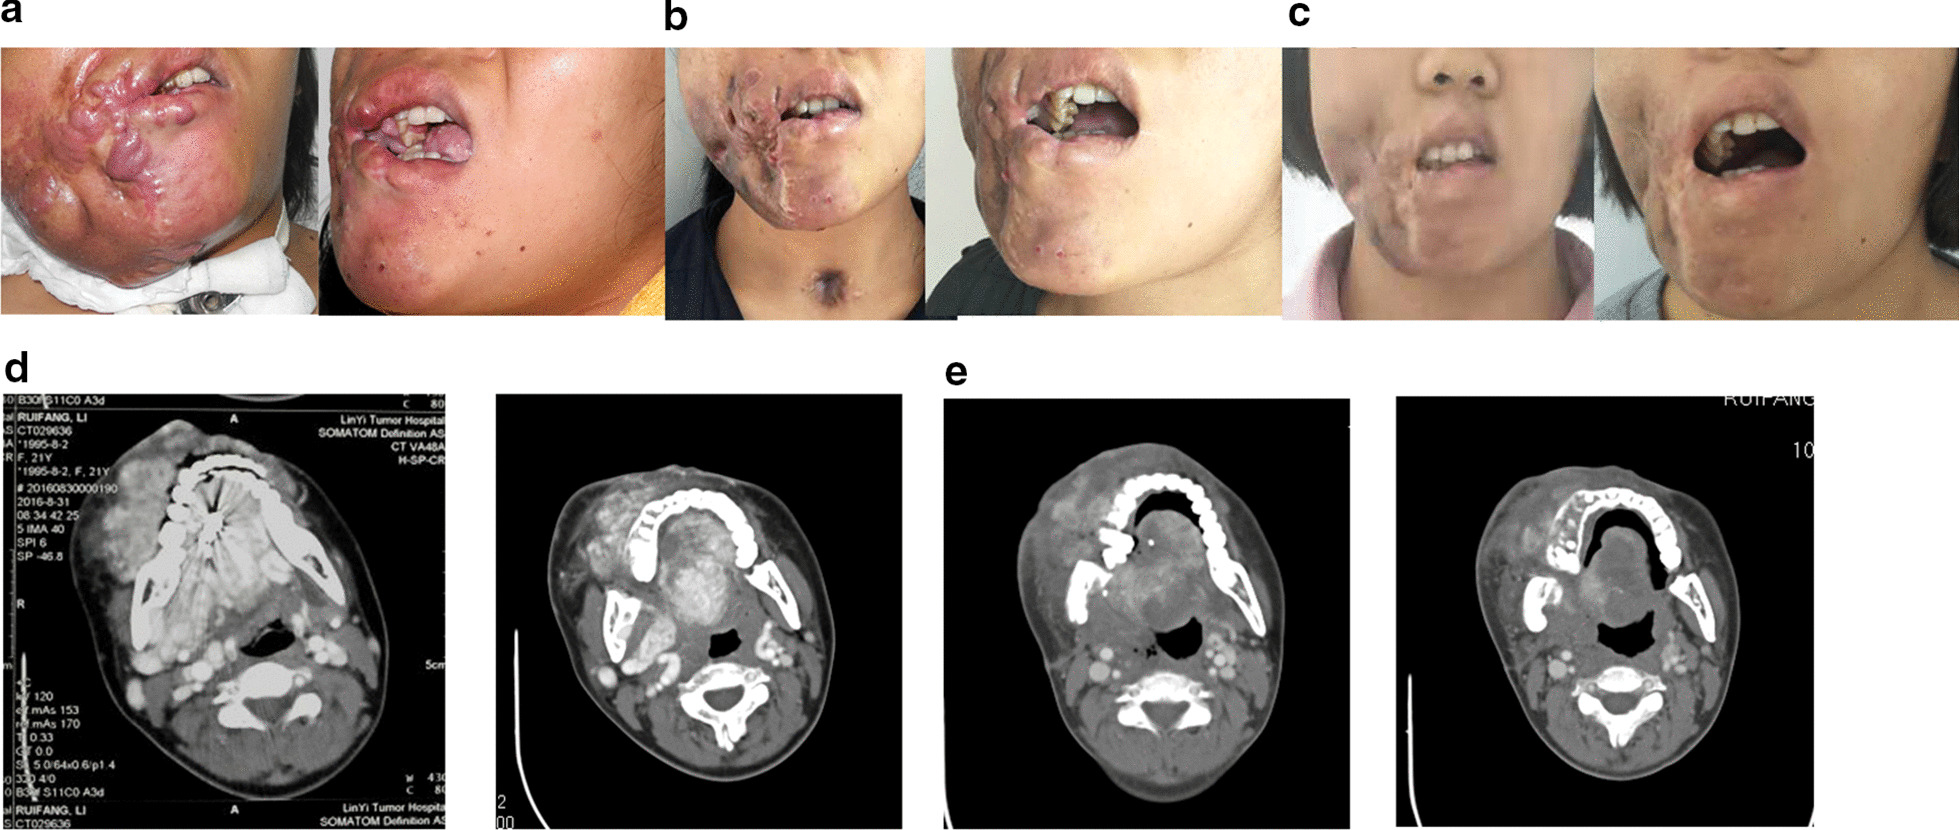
Facial lesion comparison before and after treatment. a Appearance before treatment: facial lesions have a high skin temperature, with obvious arterial pulsation, intraoral gingival thickening, tongue body thickening, and tongue body thickening. b After treatment, the facial lesions significantly reduced, the color recovered, the tongue body became smaller, and the gingival lesions disappeared. c After 2 years of treatment, the face was basically symmetrical, the skin color was close to normal, and there was no facial paralysis. d The CTA showed diffuse enhancement of right maxillofacial tongue lesions, and right neck A significantly circuitous thickening and increased. The diseased tongue filled the whole mouth. The pharyngeal cavity narrowed. e After treatment, the CTA showed low density changes in over 85% of the original tongue floor and facial enhancement lesions. Most of the abnormal blood supply arteries and collateral vessels disappeared, the diseased tongue body was significantly reduced, and the pharyngeal cavity was recovered
